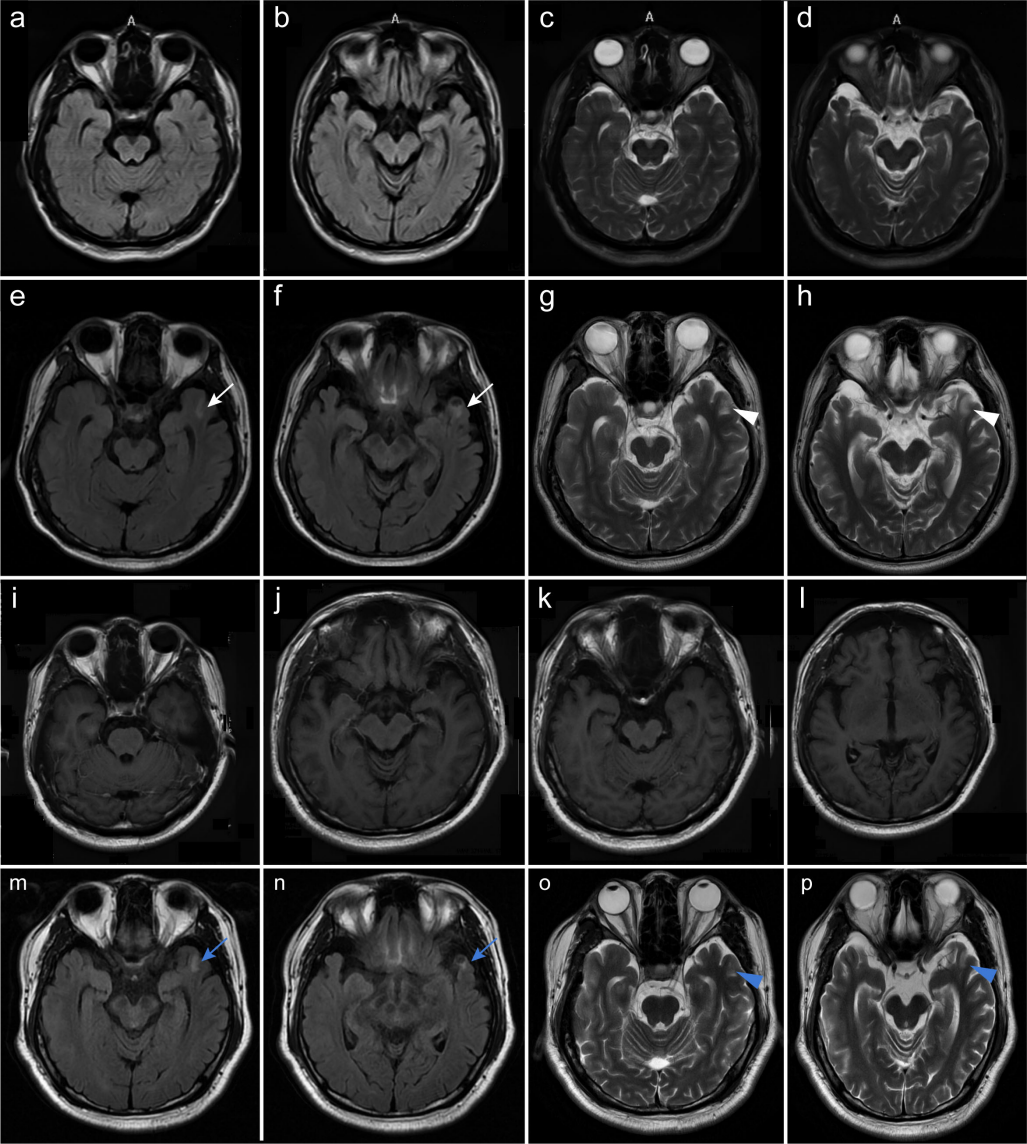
Brain MRI of the patient. Axial brain FLAIR (a, b, e, f, i, j) and T2-weighted images (c, d, g, h, k, l) are shown. No abnormalities were observed on brain MRI images (a–d) of the patient at his initial admission to our department. The patient developed a new and ill-defined patchy lesion in the left temporal lobe [(e–h); white arrows] nearly 1 month later. No significant enhancement was observed on contrast-enhanced MRI (i–l). Following 6 months of immunotherapies including plasma exchange, IVIg, and steroids, the lesion was confirmed to have shrunk [(m–p); blue arrows].
